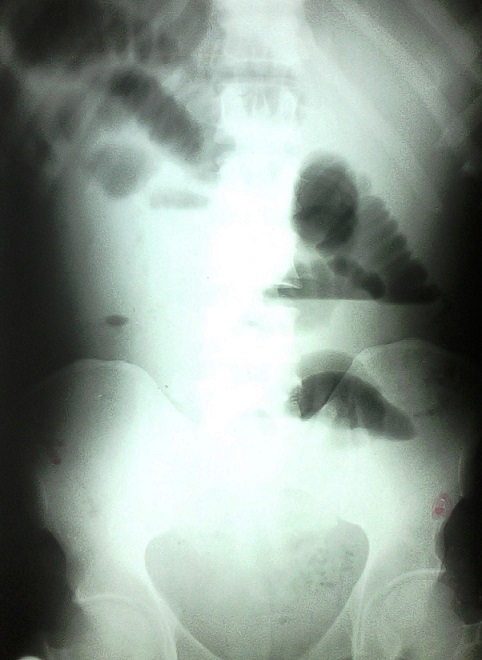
Radiographie de l'abdomen sans préparation montrant des images en faveur d'une occlusion intestinale
